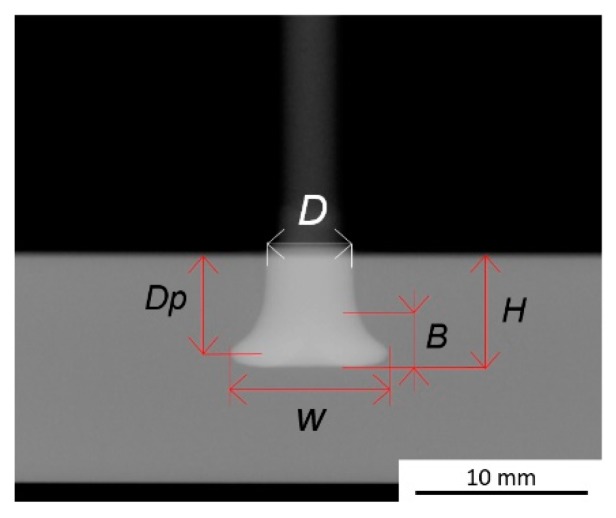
X-ray tomography joint formation measurements: rivet penetration depth (H); maximum width of the deformed rivet tip (W); depth until the maximum width of the rivet tip, or anchoring depth, (Dp); the height of the deformed rivet tip (B); and with original rivet diameter displayed (D).
